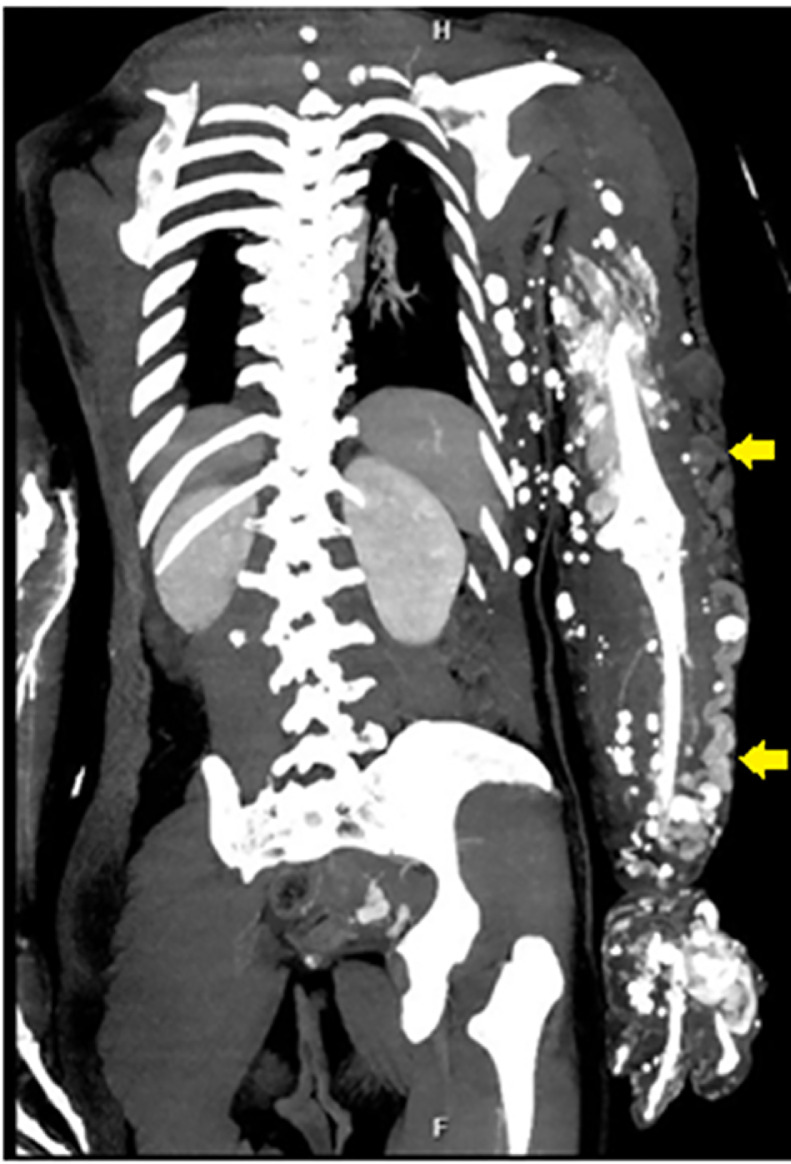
The telangiectatic superficial veins (yellow arrows) as seen in the venous phase of computed tomography scan. (Color version of figure is available online.)
